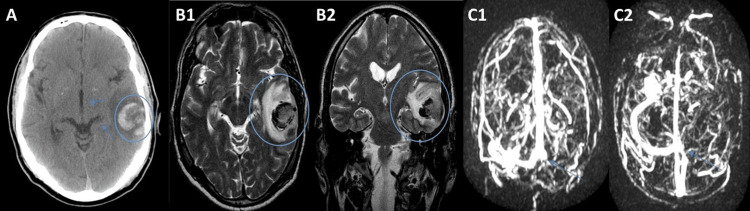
Left lateral venous sinus thrombosis (A) Cranioencephalic computed tomography (CT) shows a heterogeneous left temporal cortico-subcortical hemorrhagic lesion (circle) with a slight mass effect on the temporal horn and slight deviation of midline structures (arrows) but no brain hernias. (B) Brain magnetic resonance (MR) shows heterogeneous left temporal cortico-subcortical hematoma with surrounding edema (circle) that causes effacement of the adjacent sulci and slight deformation of the lateral ventricle - (B1) axial plane; (B2) coronal plane. (C) Brain MR angiography shows signal alteration of the lateral venous sinus on the same side with no flow in the venous angiogram obtained, suggestive of venous thrombosis (arrows) - (C1) coronal plane; (C2) axial plane.
